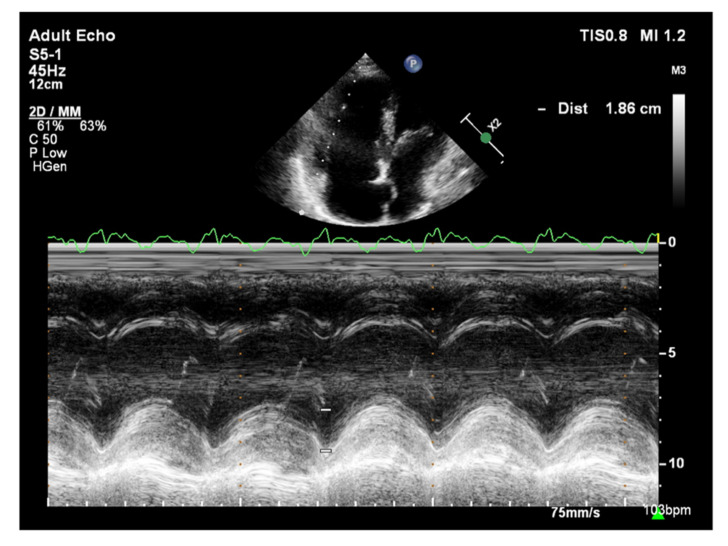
Patient with PAH, TAPSE = 1.86 cm. TTE, M-mode. PAH: pulmonary arterial hypertension; TAPSE: tricuspid annular plane systolic excursion; TTE: transthoracic echocardiography.
